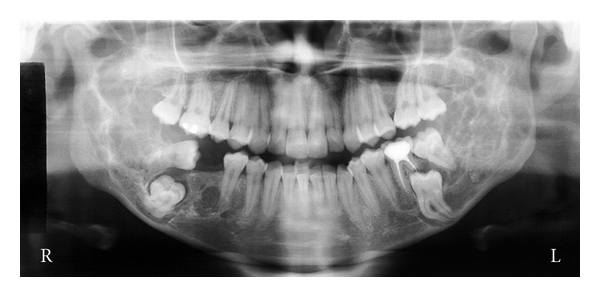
Panoramic radiograph showing bilateral growth of the lesion in the body, ramus, and coronoid process of mandible, atypical eruptions of the lower third molar, and resorption of the distal root of the left lower first molar (Gomes et al. [6]).
